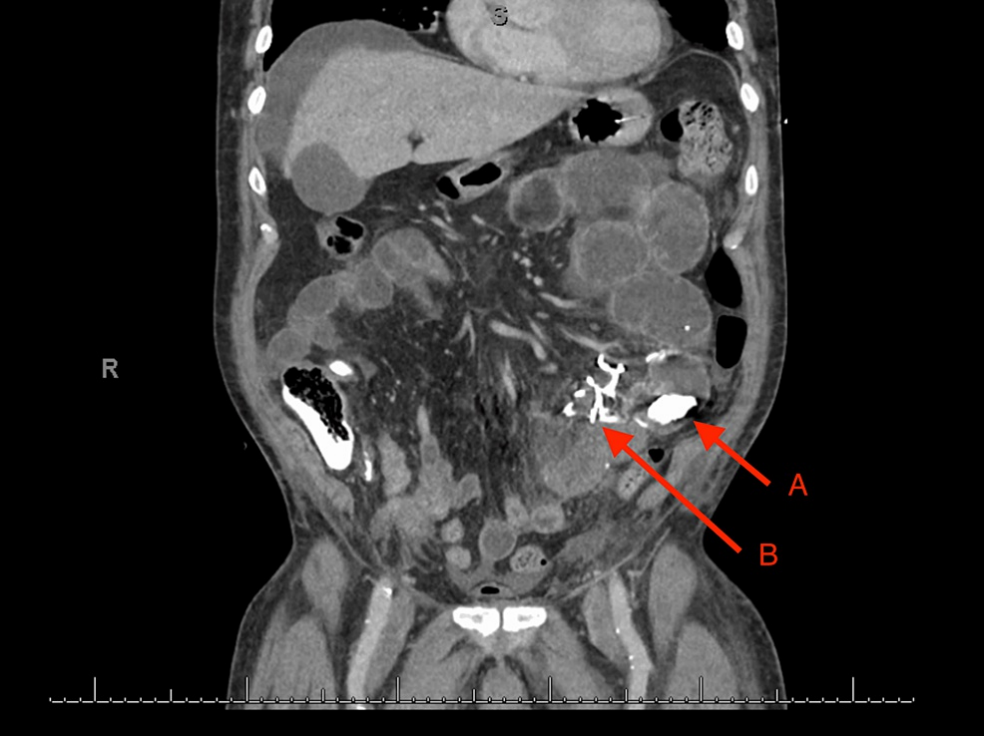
Postoperative day three arterial contrast computed tomography showing features of small bowel obstruction A: Intraluminal glue causing small bowel obstruction. B: Glue embolization into mesenteric veins.
